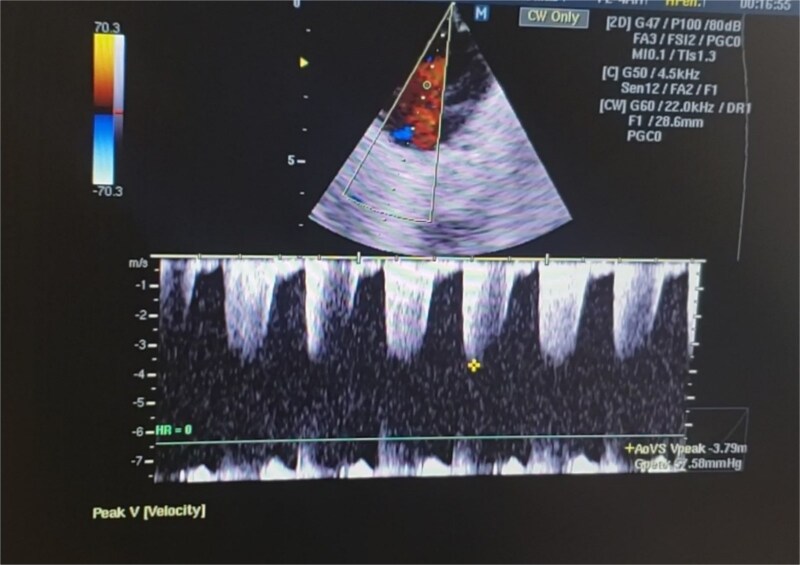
The echocardiography of the infant shows a severe PPHN with a peak pressure gradient of 67 mmHg. The combination of this disorder is associated with a worse prognosis of PRS.
